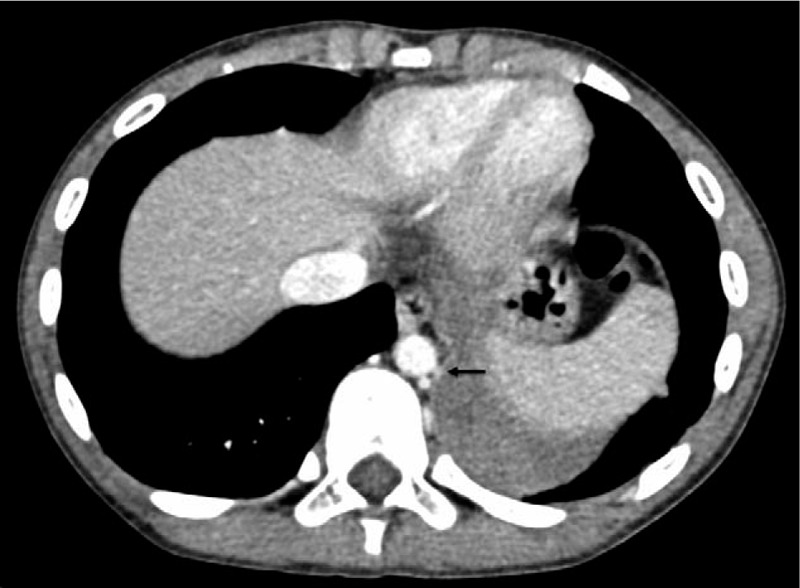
Torsion of extralobar pulmonary sequestration in a 10-year-old boy. On contrast medium-enhanced chest computed tomography, the suspected feeding artery arising from the aorta is evident (black arrow).
